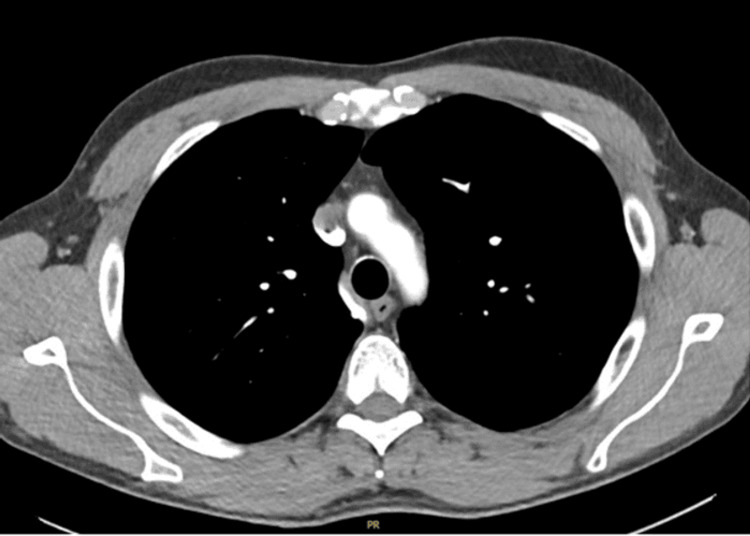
CT of the thorax (axial view) Contrast-enhanced CT of the thorax is unremarkable, with normal lung parenchyma, airways, mediastinum, and pleura.
